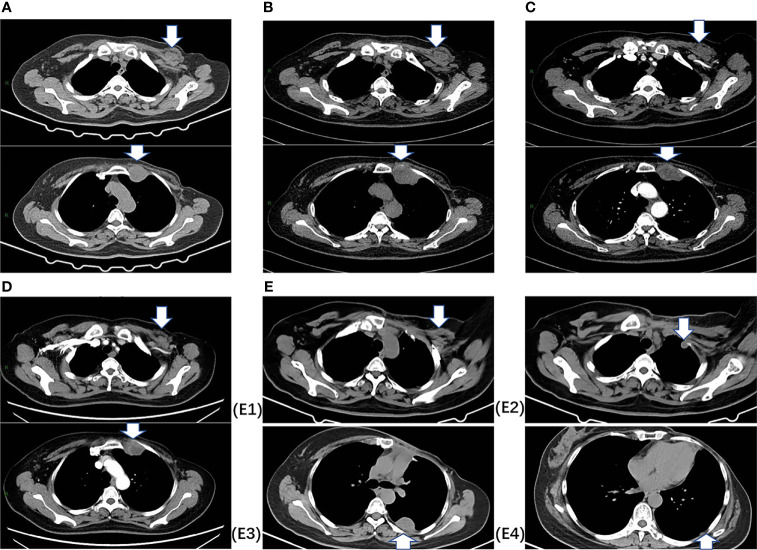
Chest CT images. (A) At baseline (July 2020), CT indicated a 2.7 cm×1.7 cm nodule in the left interpectoral region, and a 3.5 cm×3.0 cm mass in the left internal mammary area. (B) After two cycles of chemotherapy (September 2020), CT indicated stable disease (SD) with a decreased nodule size (2.4×1.5 cm) and a slight increase in the mass size (4.5×3.3 cm). (C) After four cycles of chemotherapy (November 2020), CT indicated SD with a decreased nodule size (2.2×1.2 cm) and mass (4.4×3.1 cm). (D) After six cycles of chemotherapy (February 2021), CT indicated SD with a decreased nodule size (1.5×0.7 cm) and mass (3.6×3.1 cm). (E) After surgery, E1 shows the left interpectoral node shrinkage to 1.2×0.8 cm in size, and E2, E3, E4 show appearance of multiple left pleural metastatic nodules.
